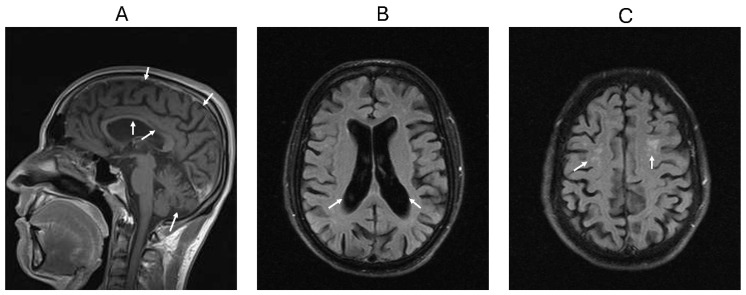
Brain magnetic resonance imaging demonstrating progressive cerebral and cerebellar atrophy in the neurologically affected sibling. (A) Sagittal T1-weighted image demonstrating diffuse cerebral and cerebellar atrophy with marked thinning of the corpus callosum (white arrows). (B) Axial T2-FLAIR image showing ventricular enlargement (white arrows). (C) Axial T2-FLAIR image revealing multifocal white matter lesions (white arrows).
